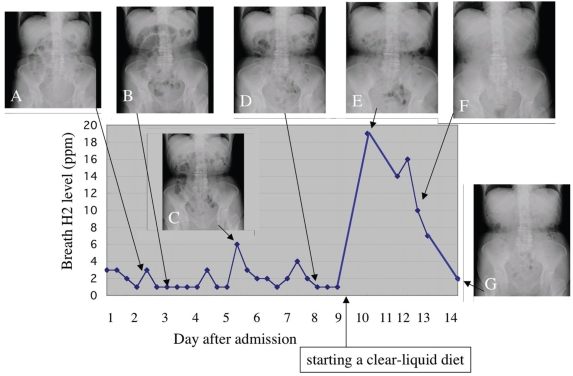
Clinical course of case 1. Changes in breath hydrogen concentration and abdominal plain radiographs on representative dates are demonstrated.
